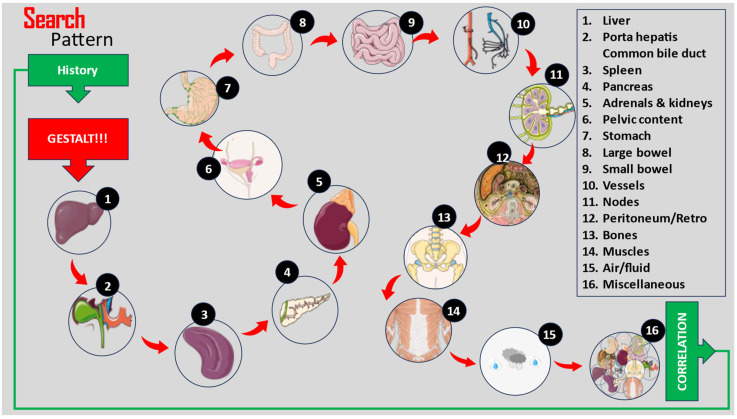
Steps for the rapid assessment of an abdominopelvic CT.
